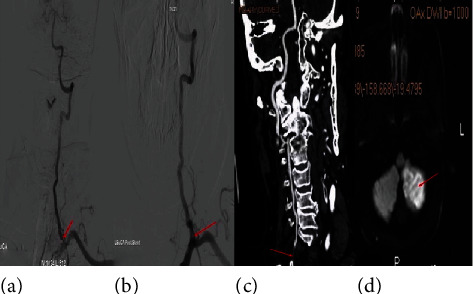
A 77-year-old male who presented with dizziness was admitted. His left subclavian arteriography shows severe stenosis of the vertebral artery ostium, and the vertebral artery ostium/subclavian artery angle was 93.9 degrees (a). After stenting of the left vertebral artery, ostium stenosis showed excellent dilatation of the lesion; then, the vertebral artery ostium/subclavian artery angle was 72.0 degrees (b). About ten months later, he was readmitted to the hospital because of dizziness reattack. His neck CTA showed left vertebral artery ostium occlusion (c). Brain MRI in DWI sequence showing left cerebellum restricted diffusion (d). Arrow in (a), stenosis site; arrow in (b), stent site; arrow in (c), occlusion site; arrow in (d), left cerebellum ischemic lesion.
